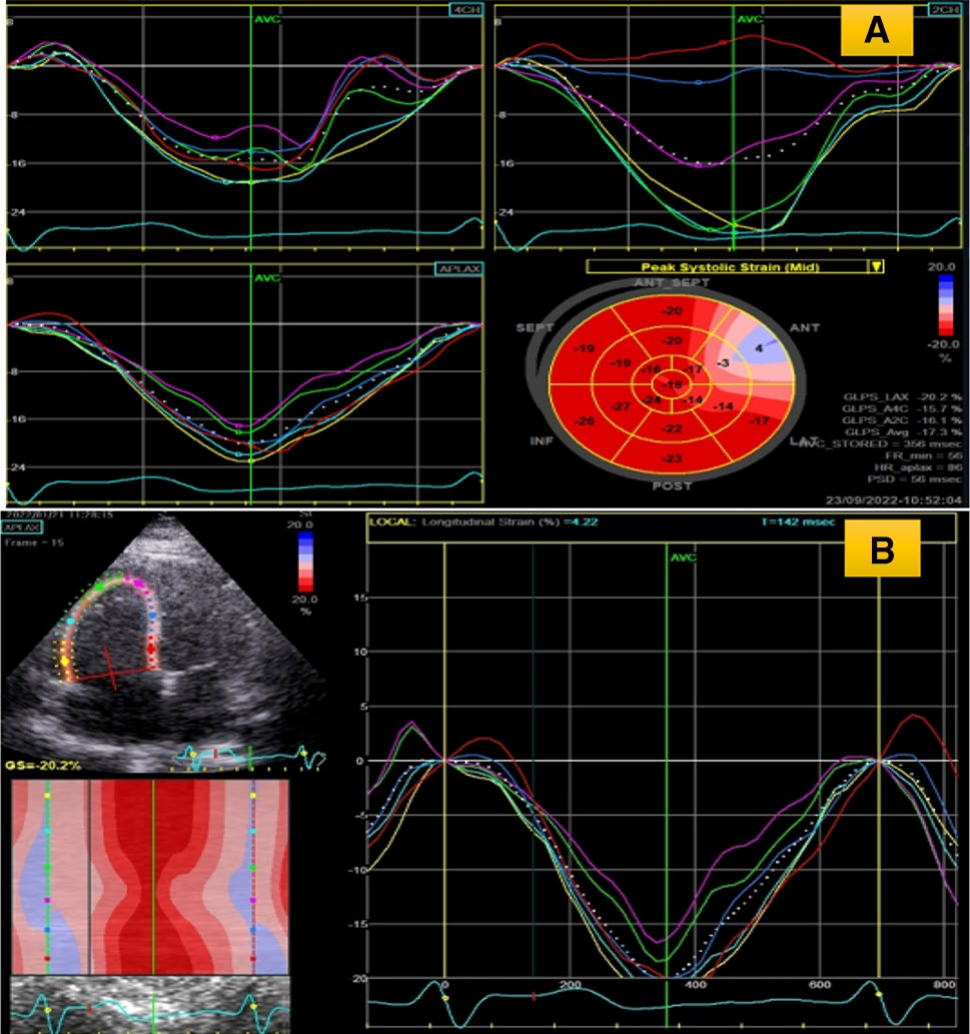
Transthoracic echocardiographic and 2D strain analysis in one of the present study’s patient with MVD (Moderate mitral stenosis and regurgitation + severe aortic regurgitation + moderate tricuspid regurgitation; (A). Tissue deformation imaging showing LV global longitudinal strain of (− 17.3%) and (B). Tissue deformation imaging showing RV strain of − 20.2%
